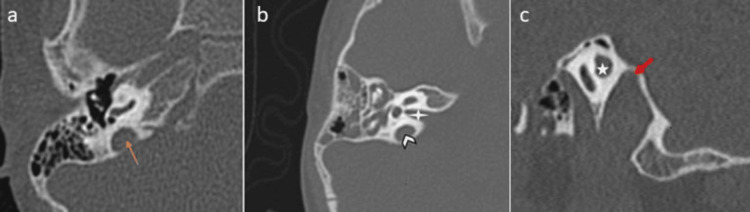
(a-b) Position of jugular bulb types (a) HRCT of the temporal bone showing the normal position of the jugular bulb (arrow), (b) axial image of HRCT of the temporal bone showing the superior part of the jugular bulb (arrowhead) seen at the level above the floor of the internal auditory canal (star), considered as a high-riding jugular bulb, and (c) reformatted sagittal plane of HRCT of the temporal bone showing the superior margin (red arrow) of the jugular bulb extending above the floor of the ipsilateral internal auditory canal (star). HRCT: high-resolution CT
